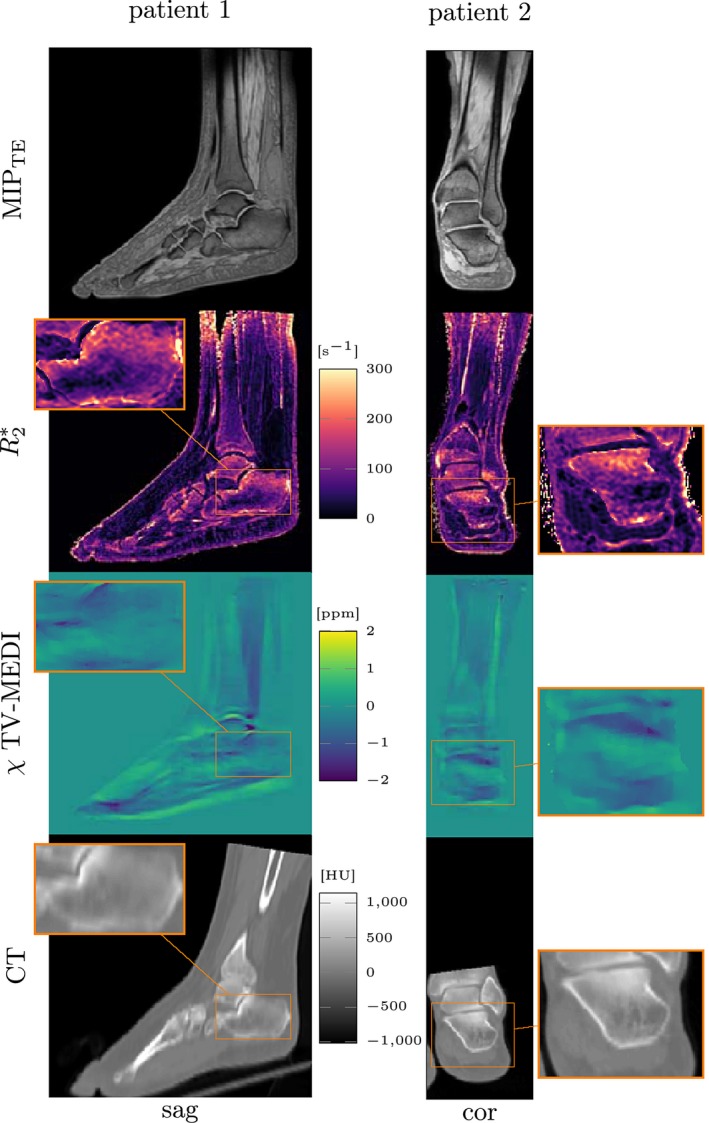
Quantitative parameter maps estimated from the MR time‐interleaved multi‐gradient echo scan compared to computed tomography (CT) available in two patient datasets. The susceptibility *χ*—estimated with an ℓ_1_‐total variation (TV) regularized morphology‐enabeled dipole inversion—depicts regions of greater trabecular density (high CT attenuation) with more diamagmetic values. An extended version of this Figure including all orientations is available as Supporting Information Figure S4
